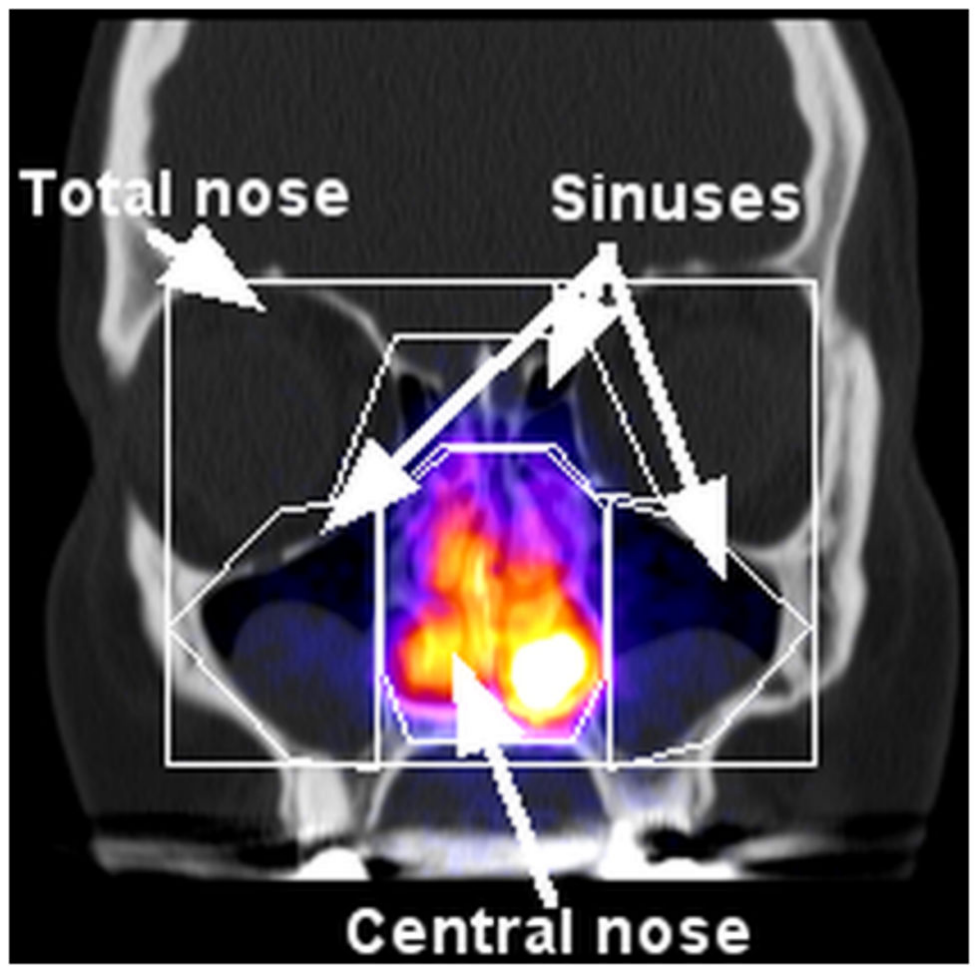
Definition of regions of interest (ROI’s) in anterior gamma camera images. Superposition of the anterior gamma camera image with a representative coronal CT slice of a CRS patient before FESS and definition of the total nasal, central nasal and sinus ROI’s.
